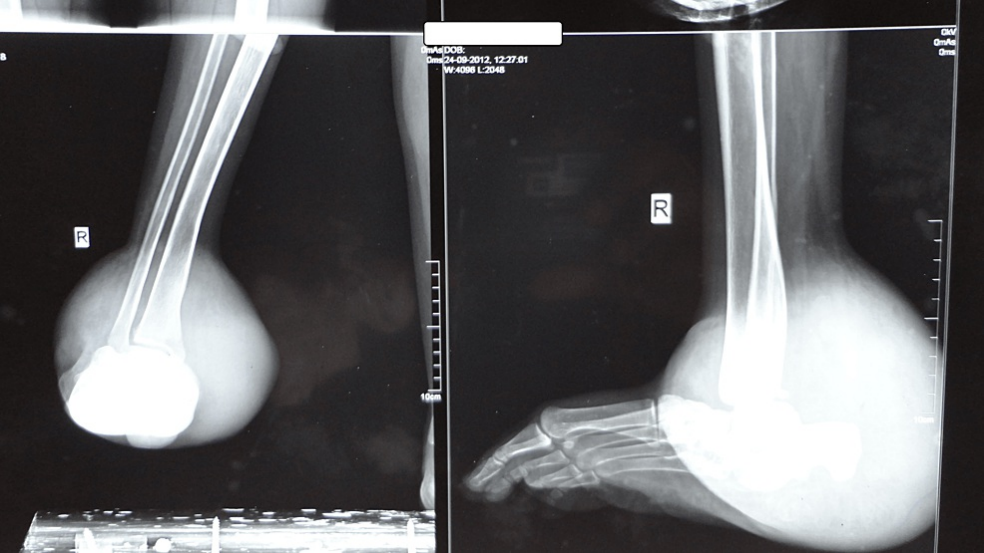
X-ray of the right ankle (oblique and lateral view) shows soft tissue shadows around the ankle joint with anterolateral bowing of the tibia.
